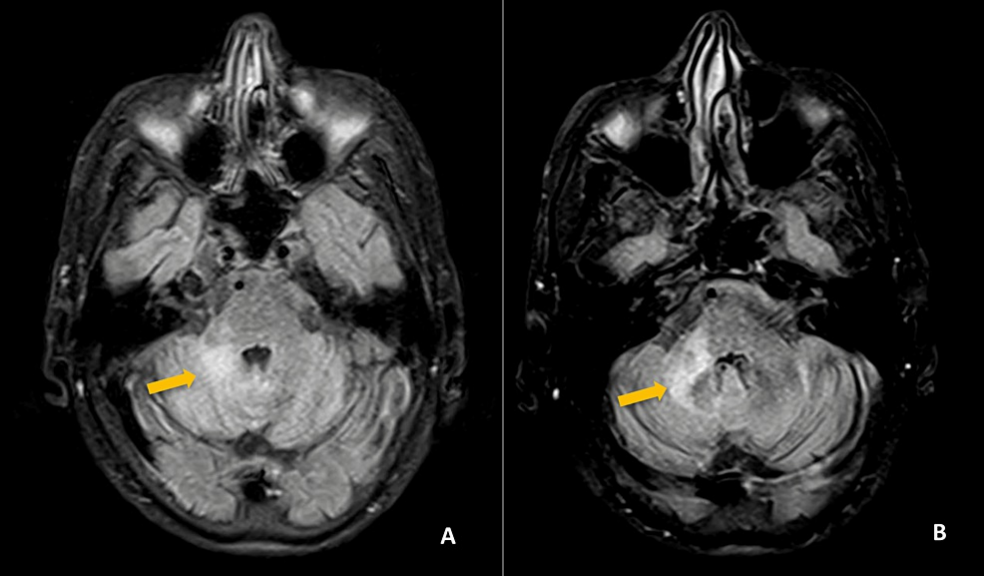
First brain MRI two weeks after symptom initiation, transverse T2/FLAIR sequence (A and B) with hyperintense lesion on the right cerebellar peduncle (yellow arrows). FLAIR: fluid attenuated inversion recovery
